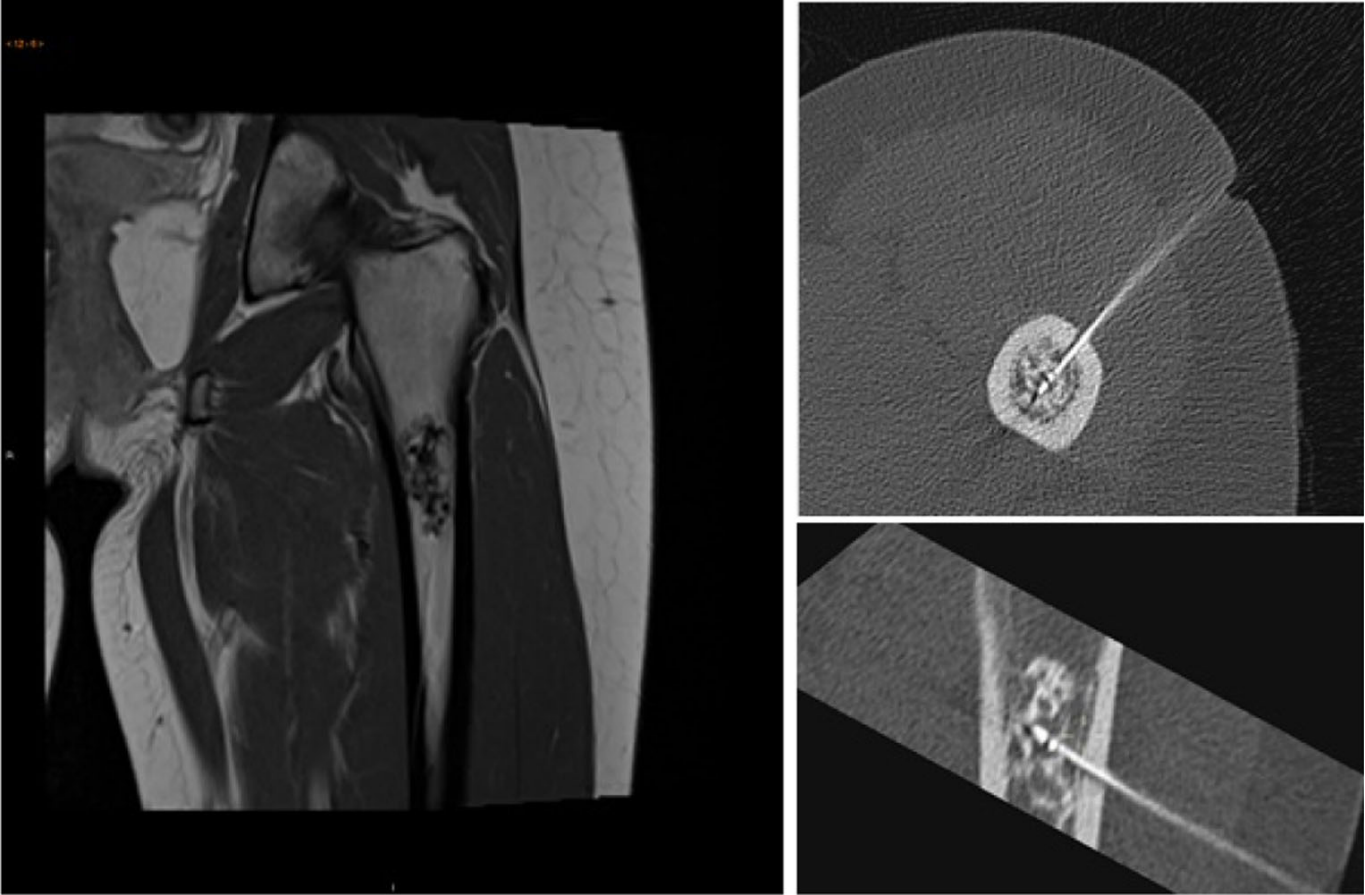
a Representative MRI of an atypical cartilaginous tumour (ACT) in the proximal femur. b Transverse and c coronal images of CT-guided radiofrequency ablation (RFA) of the same tumour
